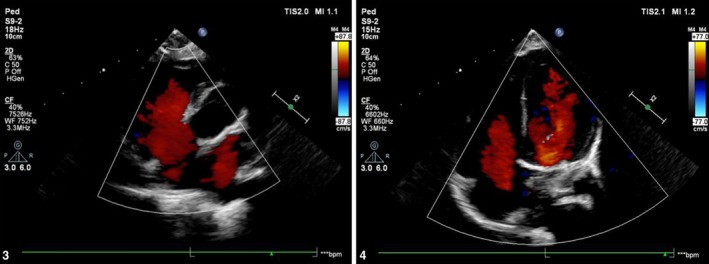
Postoperative echocardiogram confirming successful VSD closure and normal ventricular function.
